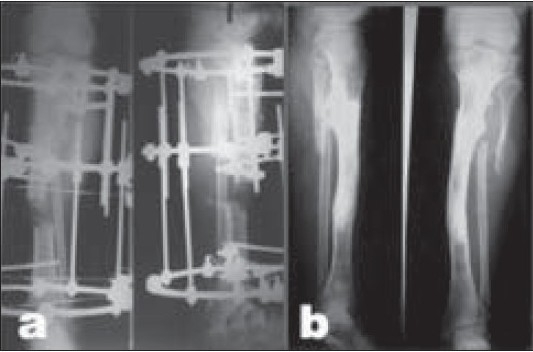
(a) Radiograph of the same leg (lateral and anteroposterior view) with Ilizarov fixator after conversion from monolateral fixator with insertion of bone graft. (b) Followup radiograph (lateral and anteroposterior view) shows consolidation of the nonunion and limb lengthening of 6 cm.
